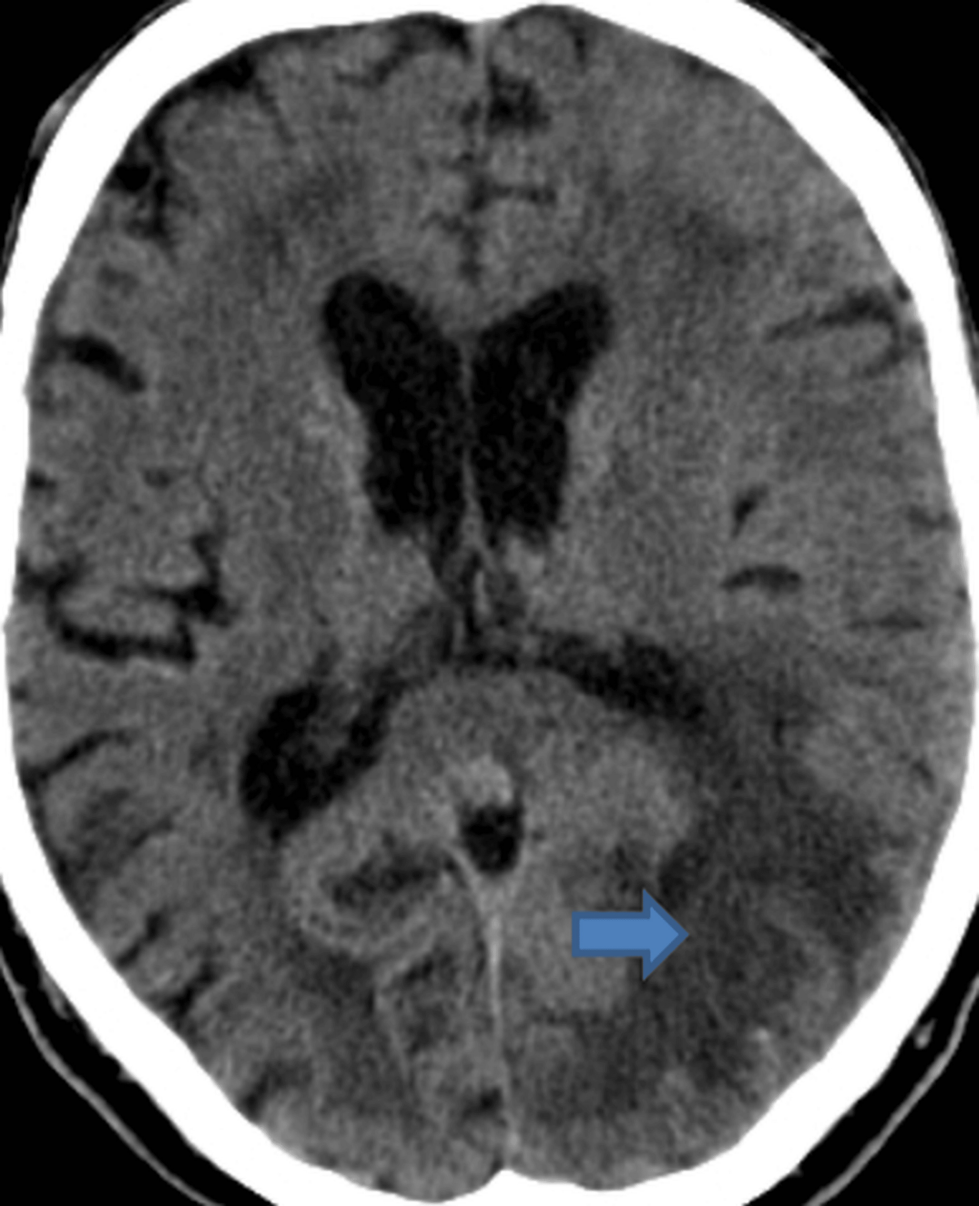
CT head of the patient The arrow is pointing to a region of hypoattenuation in the parieto-occipital region.
